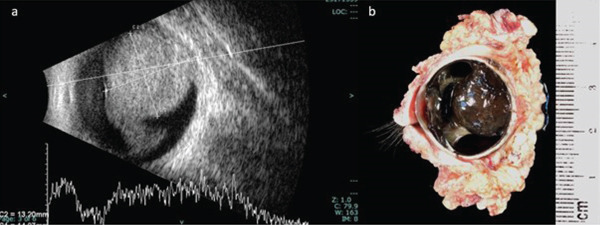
(a) Ocular sonography showing mass attached to choroid at the superior section of vitreous cavity. (b) Sagittal section of left eyeball with periorbital soft tissue, and both eyelids demonstrates an intraocular, dark tan mass.
